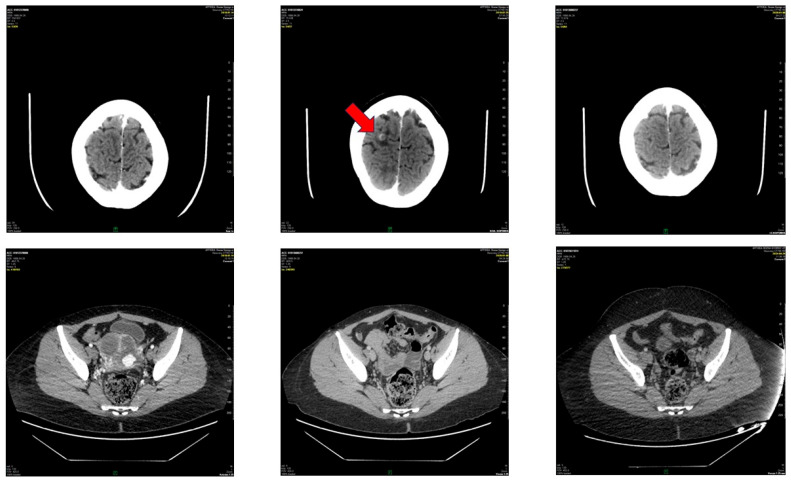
Appearance of brain metastasis can be seen (red arrow), and its complete regression after stereotactic radiotherapy (upper line). CT image of the pelvis with uterus affected by choriocarcinoma before surgery and complete regression of the disease after surgery, multiagent chemotherapy and 62 cycles of pembrolizumab. GE Optima^TM^ 660 (GE Health Care, 500 West Monroe Street, Chicago, IL 60661, USA) was used.
